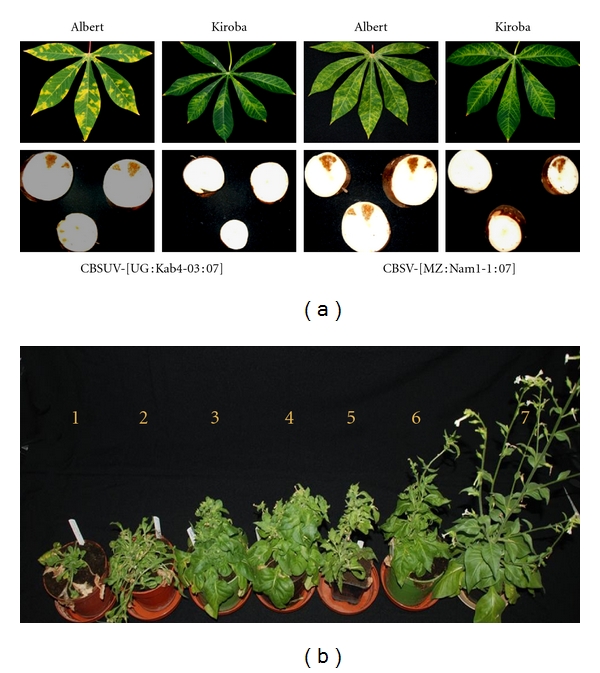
(a) Typical leaf and root symptoms expressed by the CBSUV-[UG:Kab4-3:07] and CBSV-[MZ:Nam1-1:07] in varieties Albert and Kiroba. (b) Typical symptoms observed on *N. clevelandii* plants 5-6 weeks after inoculated with sap extracted from infected cassava plants. 1 = CBSV-[MZ:Nam1-1:07]; 2 = CBSV-[TZ:Nal3-1:07]; 3 = CBSV-[TZ:Kib10-2:03]; 4 = CBSV-[TZ:Zan6-2:08]; 5 = CBSUV-[KE:Mwa16-2:08]; 6 = CBSUV-[UG: Kab4-3:07]; 7 = healthy control plant.
